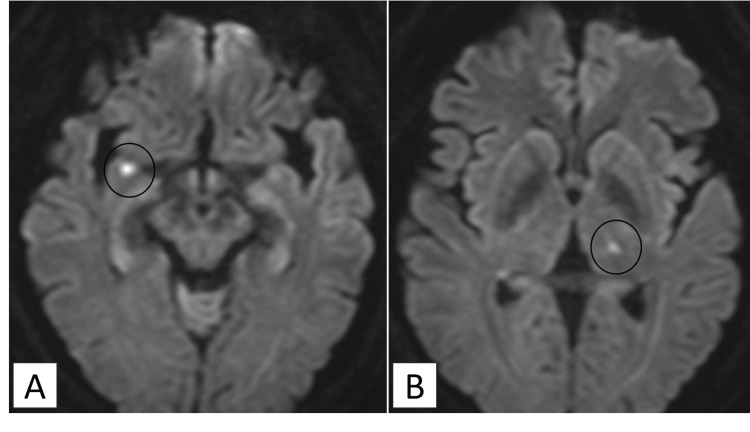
Case 1: Axial diffusion-weighted images (DWI) on day three Panel (A) shows a hyperintense lesion in the right lateral amygdala (circle). Panel (B) shows a hyperintense lesion in the left thalamus (circle).
